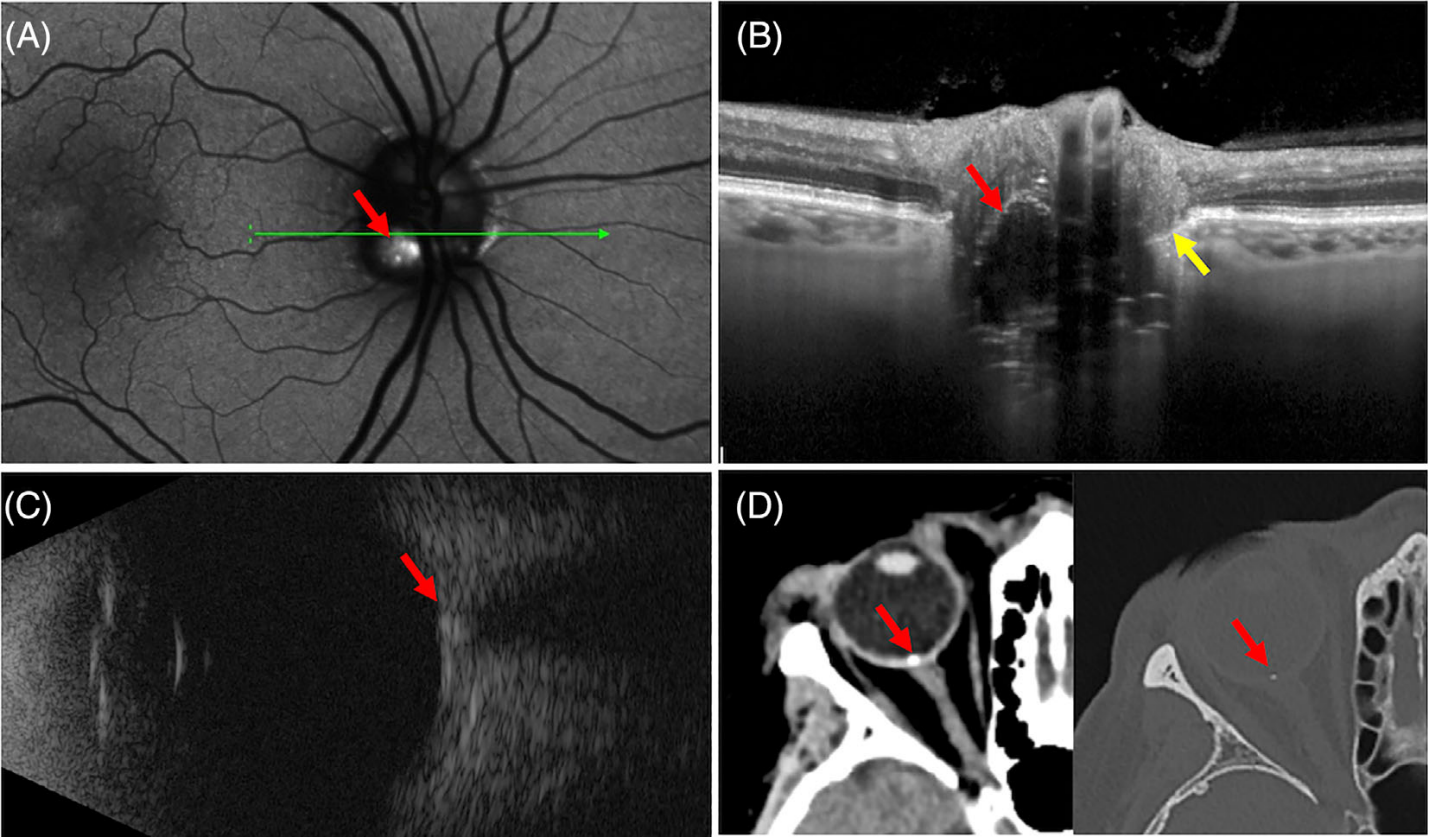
Optic disc drusen (ODD) in a 78‐year‐old female with macular telangiectasia. Fundus autofluorescence showed the typical hyperautofluorescence of the ODD at the disc and foveal hyperautofluorescence due to loss of xanthophyll pigment (A). Corresponding OCT through the ODD showed a deep hyporeflective structure with a hyperreflective superficial border (red arrow) and accompanying PHOMS (yellow arrow, B). The optic nerve head was hyperechogenic on B‐scan ultrasound (C) and bright on computed tomography in the bone window sections indicating calcium (D).
